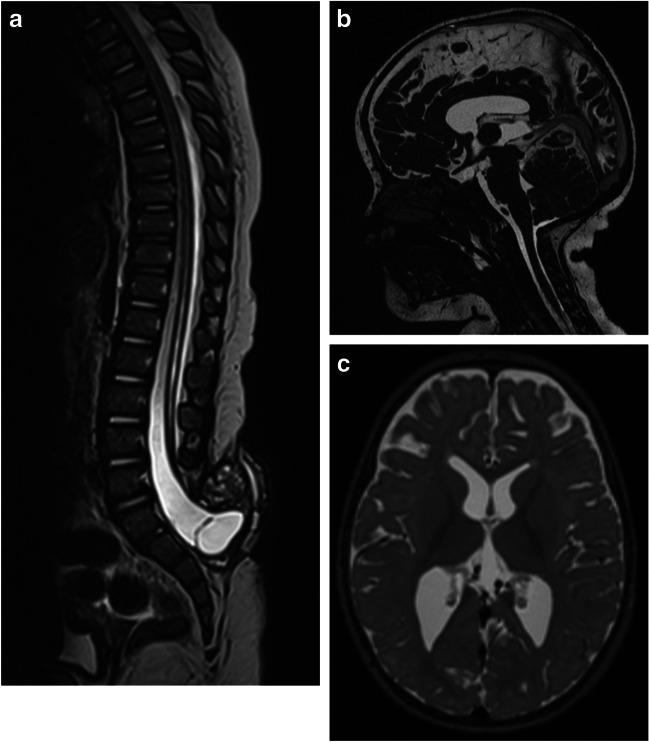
MRI taken 8 months after MMC repair showed A tethered spinal cord with tiny syringomyelia from Th11 through L5. B Although the tectum still was beaked and the posterior cranial fossa was small, there was no longer any visible herniation of vermis into the foramen magnum, and the position of pons and medulla oblongata was normalized, with adequate CSF space around the medulla oblongata in the foramen (3D CISS). C Supratentorial ventricles were slightly asymmetrical and only moderately enlarged (T2WI)
